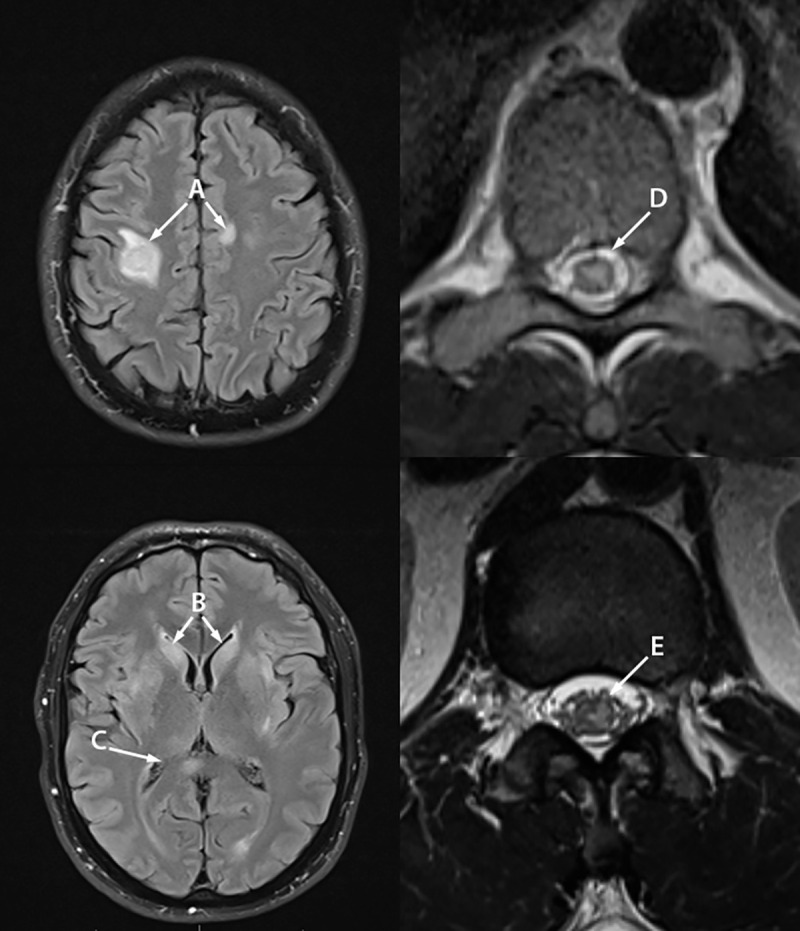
Magnetic resonance imaging of the brain, thoracic spine, and conus medullaris of patient A with monkeypox-associated encephalomyelitis showing abnormal T2/fluid attenuated inversion recovery signal in the right frontal and left frontal lobes (A), bilateral basal ganglia (B), bilateral medial thalami and right splenium (C), central thoracic spinal cord (D), and gray matter of the conus medullaris (E) — Colorado, July–August 2022 Photos/Daniel M. Pastula.
